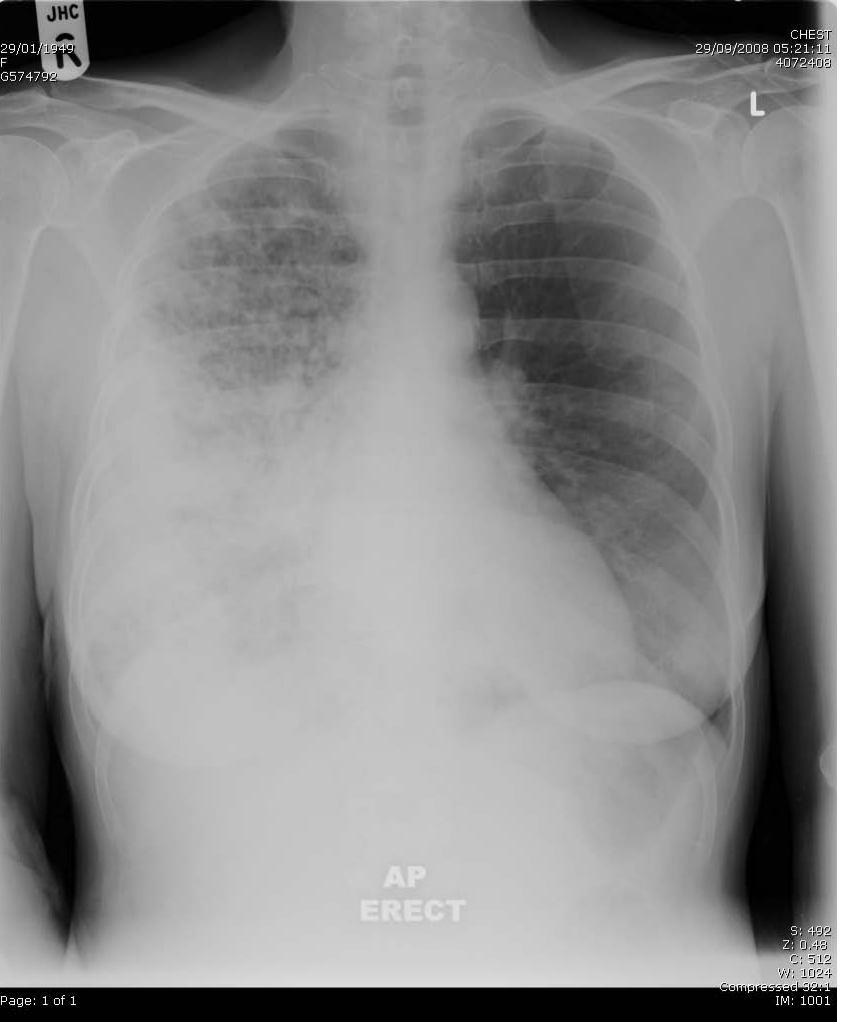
Chest X-ray on admission which showed right lower lobe consolidation'.
